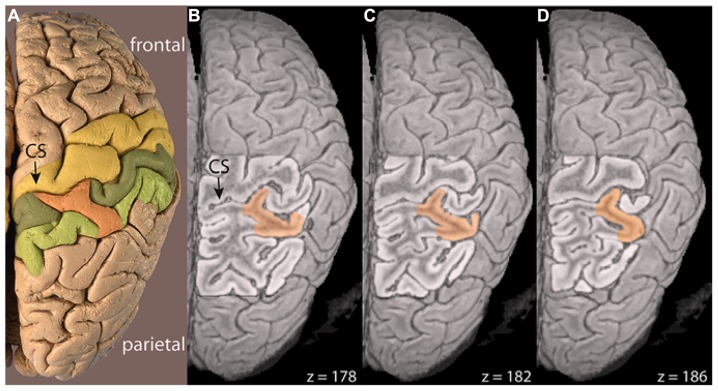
(A) Photograph and (B–D) cross-sectional MRI views of the right-hemispheric brain of C. H. Fuchs cutting into the depth of the central sulcus (CS, arrow). *z* = z-coordinate in mm, post-central gyrus = dark green, parietal gyrus = light green, bridges = orange.
